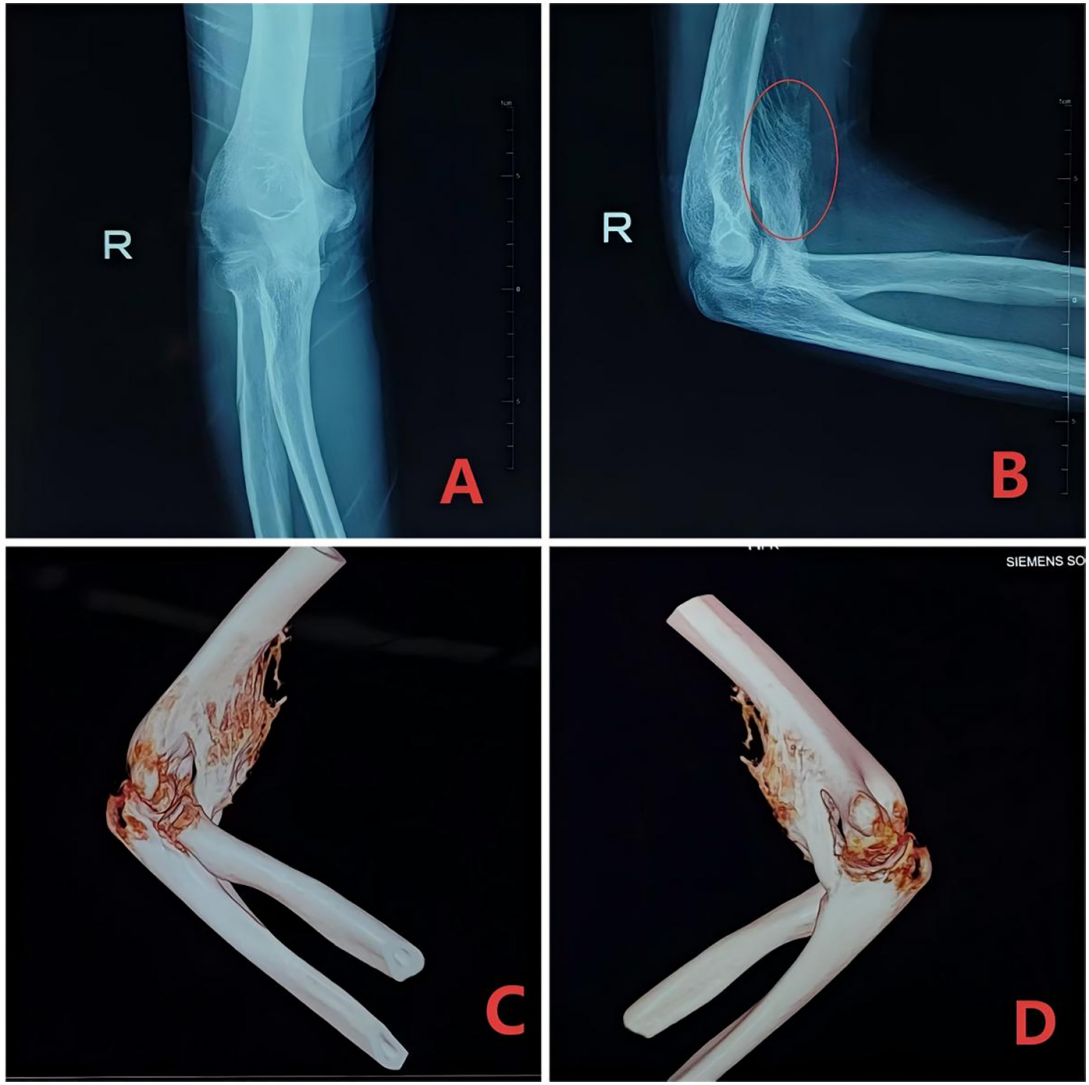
A 24-year-old male presented with right elbow stiffness for 4 months. Heterotopic ossification was observed adjacent to the right ulnar coronoid process. The articular relationships were approximately normal, with no significant widening or narrowing of the joint space. (A,B) Anteroposterior and lateral x-ray films. (C,D) Three-dimensional imaging of the right elbow joint.
